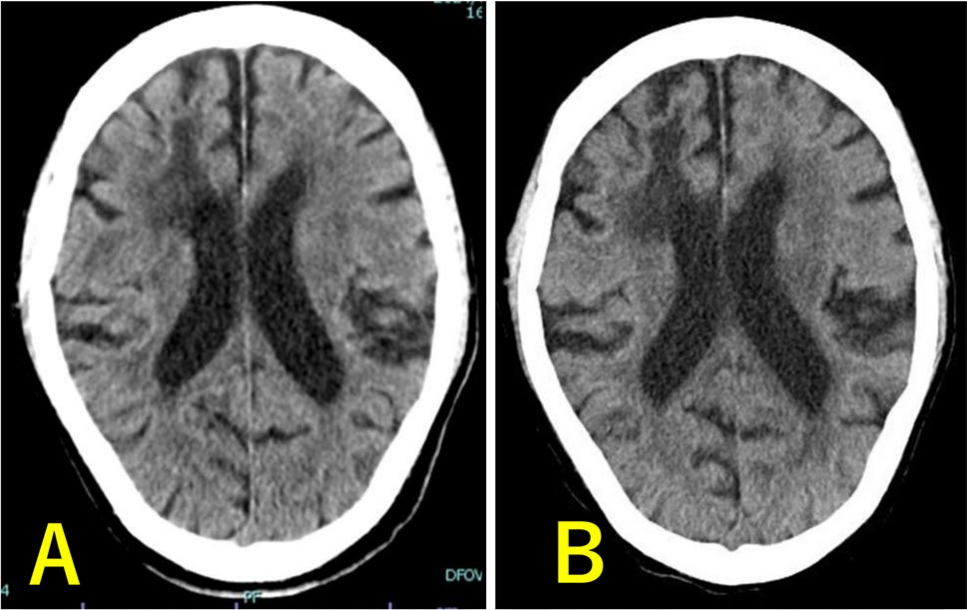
Preoperative CT and CT on POD9. A Preoperative CT. B POD9 CT. Showing resolution of edema and sulcal restoration. CT: computed tomography. POD: postoperative day
